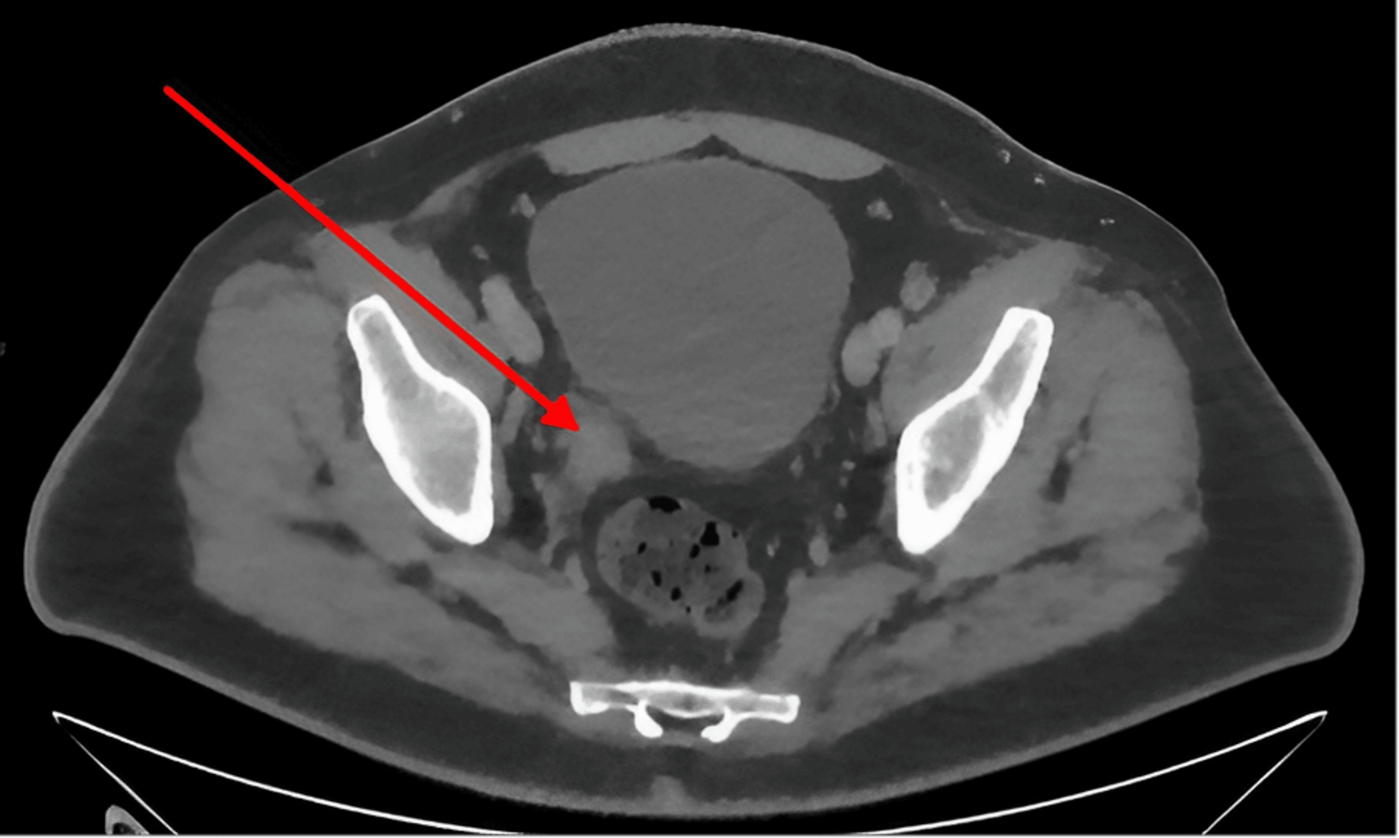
Cross-sectional CT image showing soft tissue mass at distal right ureter CT, computed tomography
